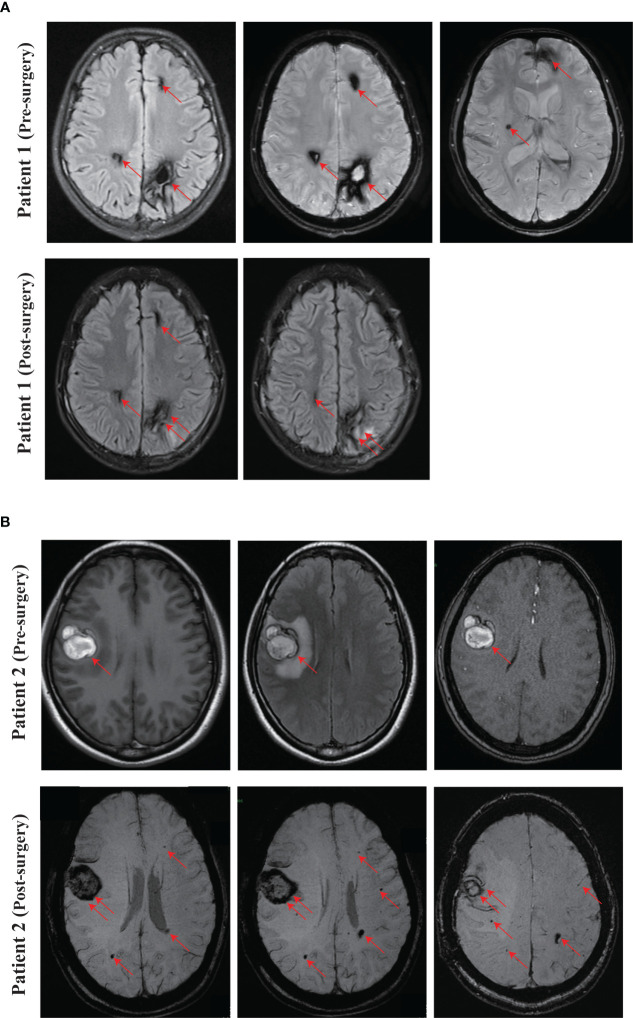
Representing images of CCM. (A) SWI images of the proband (Patient 1, IV-1) at pre-surgery and 3 months after surgery. (B) Pre-surgery cerebral MRI images of the proband’s mother (Patient 2, III-4), and SWI images at one month after surgery. Red arrow, CCM lesion. Double red arrows, surgical site.
